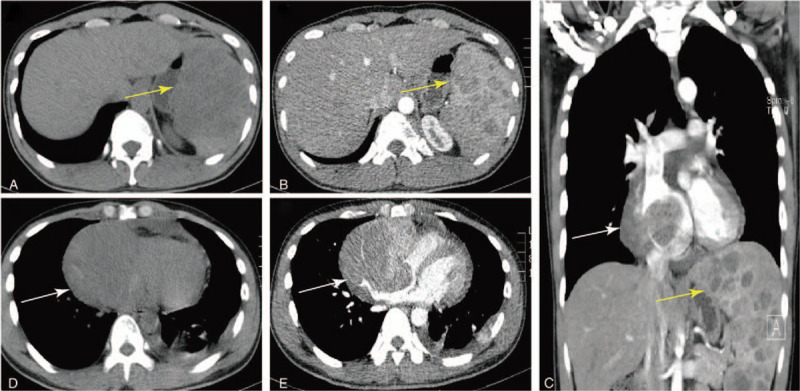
CT images (A, yellow arrow) showed splenomegaly with multiple low-density nodules with ring enhancement in contrast-enhanced CT imaging (B, C, yellow arrow). A large irregular mass was also found in the right superior mediastinum with heterogeneous density (D, white arrow) and enhancement (C, E, white arrow). CT = computed tomography.
